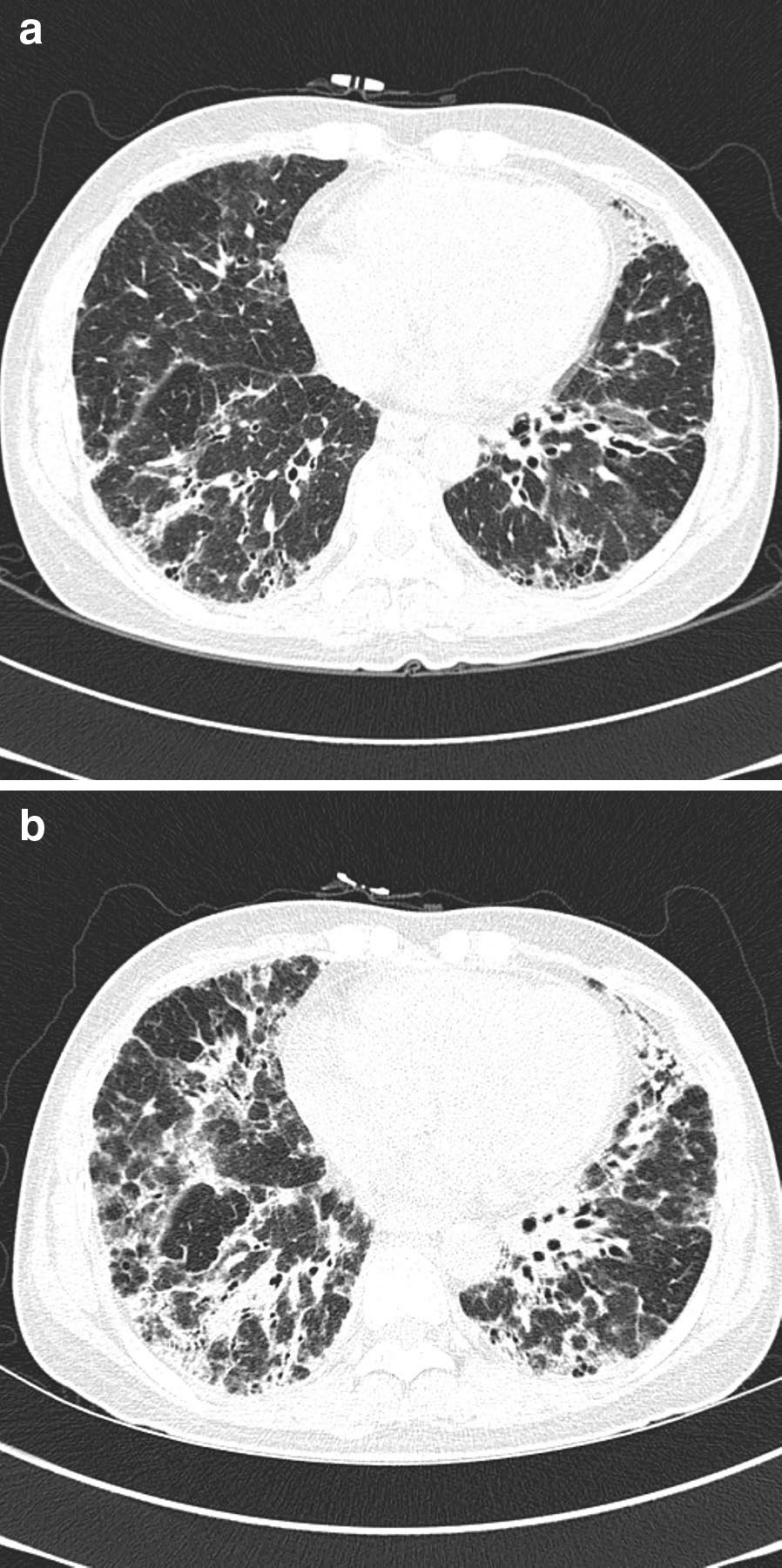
High-resolution computed tomography (HRCT) of lung. a HRCT (1 year ago) showed traction bronchiectasis and consolidation in bilateral subpleural and lower lung zone. b Recent HRCT showed an increased extent of coarse reticulation, ground glass opacity with traction bronchiectasis in bilateral subpleural and lower lung zone. Increased extent of coarse reticulation, ground glass opacity with traction bronchiectasis in bilateral subpleural and lower lung zone
